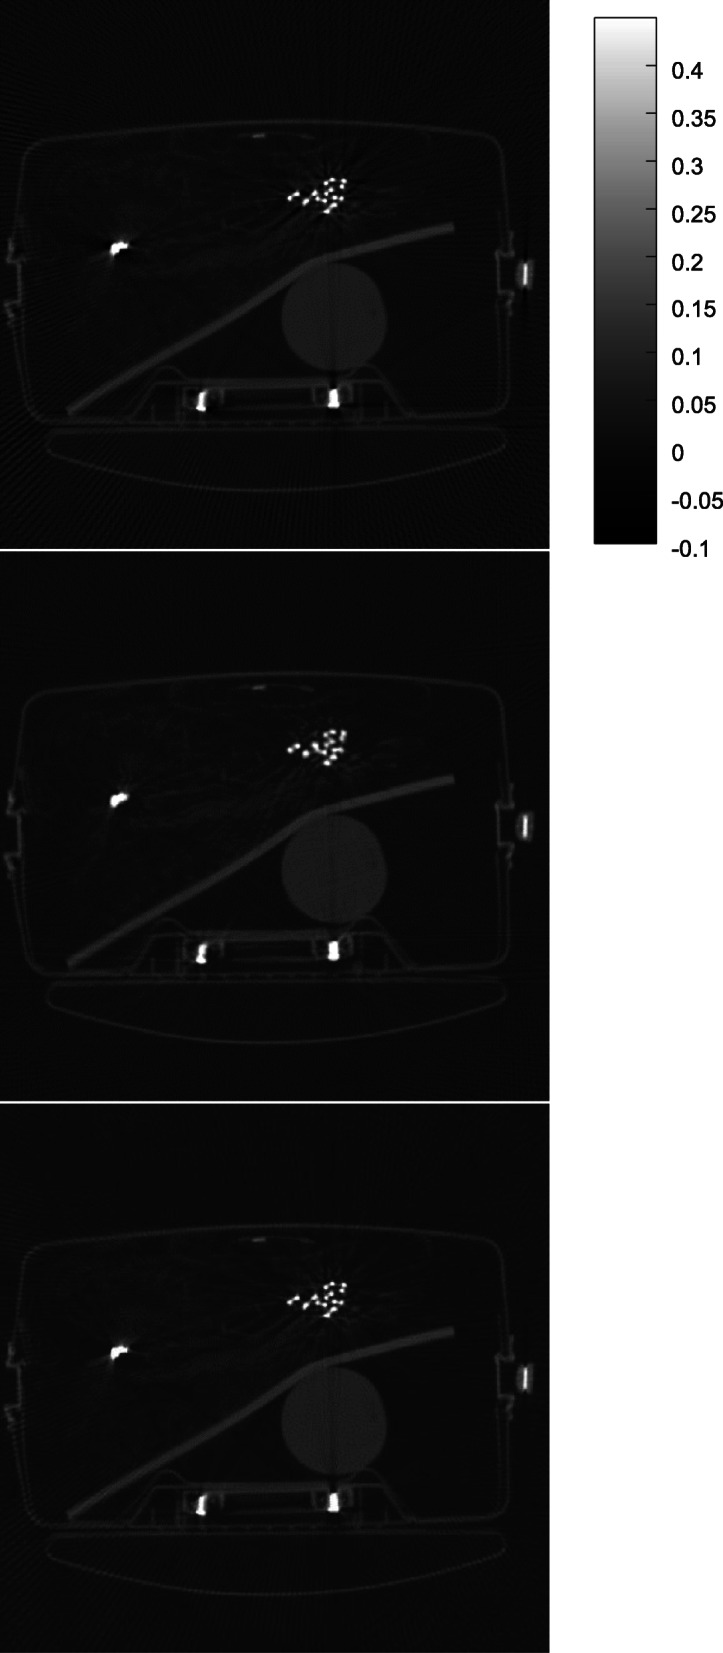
CT image reconstruction of airport bag #1. Top: the raw FBP reconstruction; Middle: the reconstruction using the proposed algorithm; Bottom: the raw FBP reconstruction with all negative pixel values replaced by zeros
